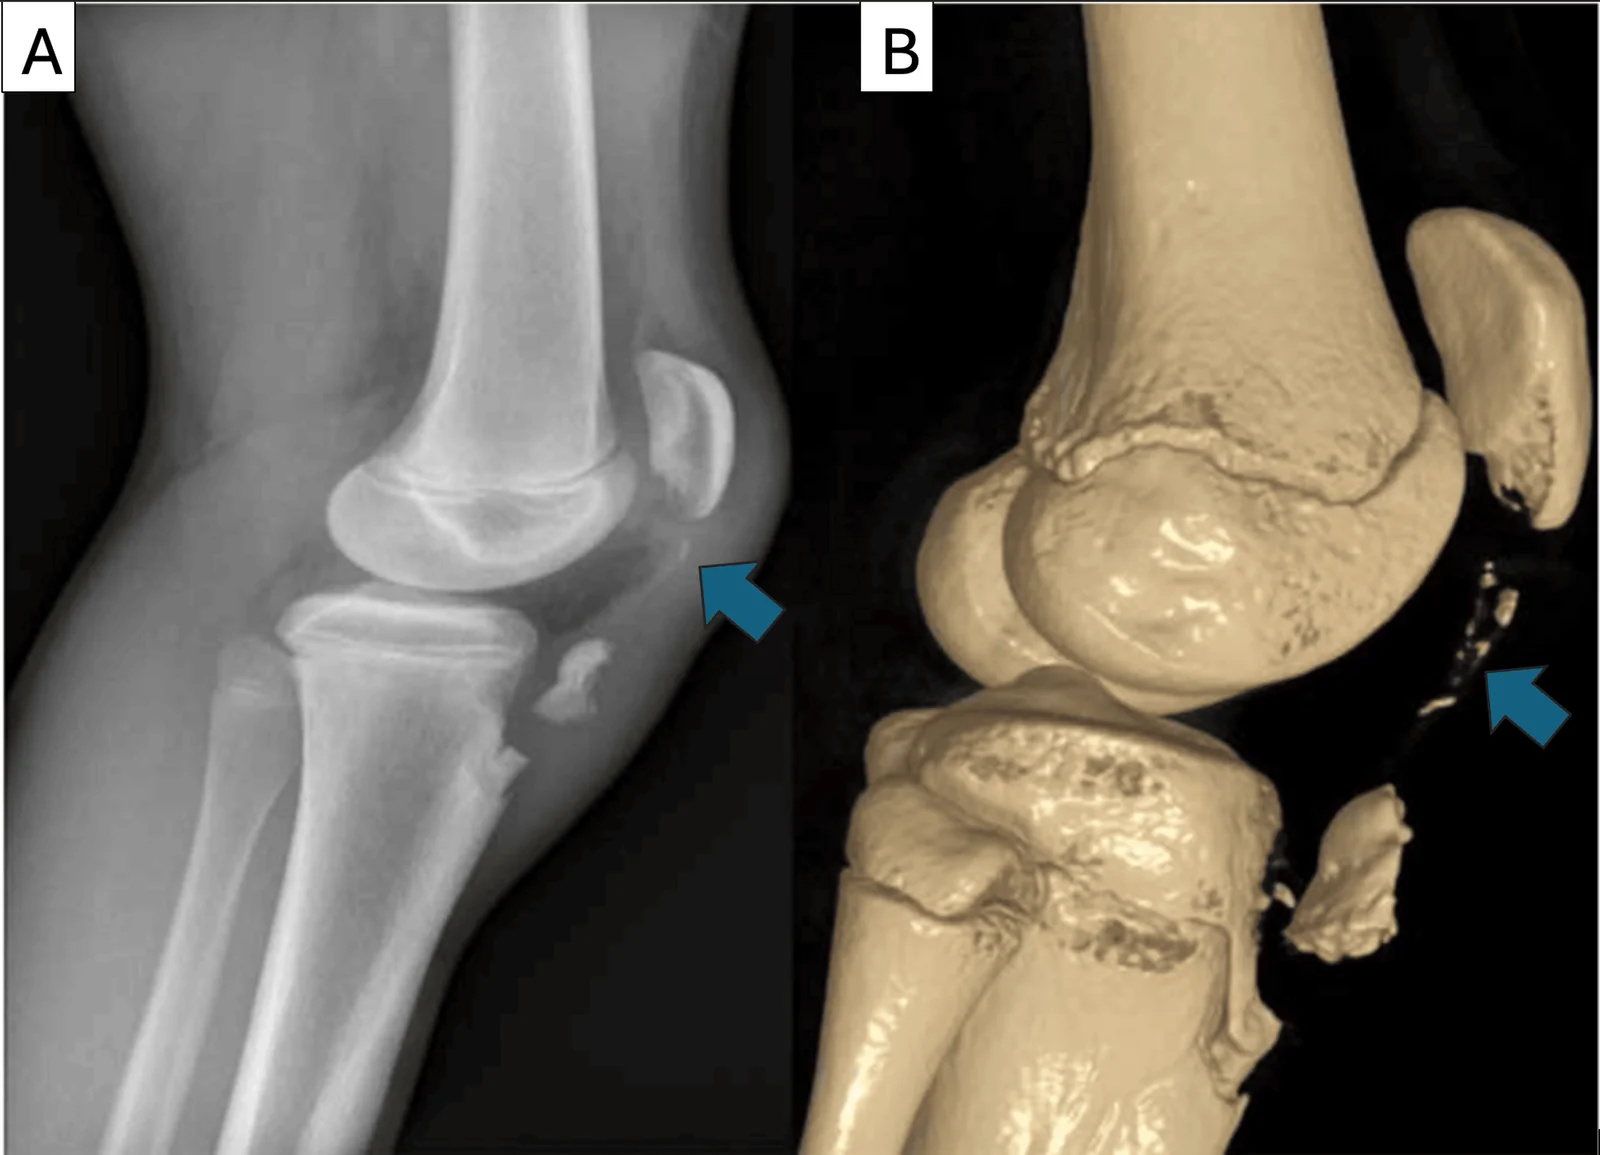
Preoperative lateral radiograph and three-dimensional computed tomography reconstruction of case 1. (A) Lateral radiograph demonstrating an Ogden type IIB tibial tubercle fracture with complete rotational displacement of the fragment. Small osseous fragments within the patellar tendon region (arrow) are consistent with the sign described by Kramer et al. [5] as a clue to associated patellar tendon avulsion. (B) Three-dimensional CT reconstruction confirming complete fragment rotation and the small osseous fragments within the patellar tendon region (arrow).
